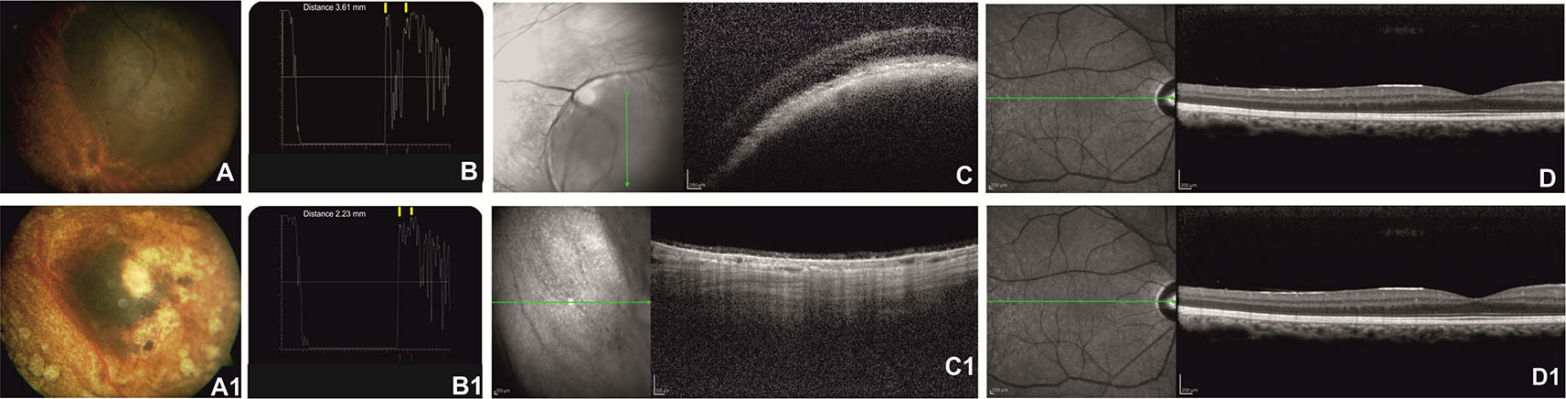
Right eye of a 57-year-old patient affected by choroidal melanoma before ruthenium-106 brachytherapy (top row). Color fundus image shows an elevated and yellow lesion in the nasal mid-peripheral of the retina (A). At A-scan ultrasound, the lesion presents low reflectivity. The yellow arrows over the peaks of the two high and perpendicular spikes, as shown in the echogram, indicate the maximum lift of 3.61 mm (B). Spectral domain–optical coherence tomography (SD-OCT) B-scan over the lesion revealed a highly reflective band within the choriocapillaris layer with posterior shadowing (C). SD-OCT B-scan shows no alteration of the retinal layer architecture in the macular region (D). Color fundus image shows the same tumor after ruthenium-106 brachytherapy (A1). At A-scan ultrasound, the lesion presents high reflectivity with a tumor thickness of 2.23 mm (B1) confirmed also by the OCT B-scan (C1). OCT B-scan shows no alteration of the retinal layers architecture in the macular region (D1).
